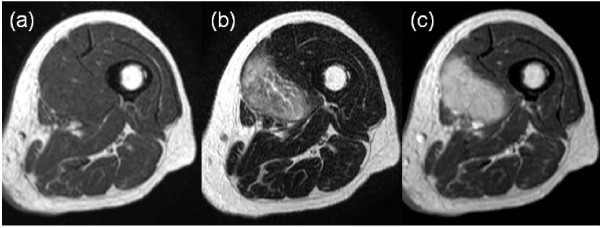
A) MRI of the thigh showing iso signal intensity in a T1-weighted image. B) Heterogeneous high signal intensity in a T2-weighted image. C) Diffuse enhancement in a post-contrast T1-weighted image.
